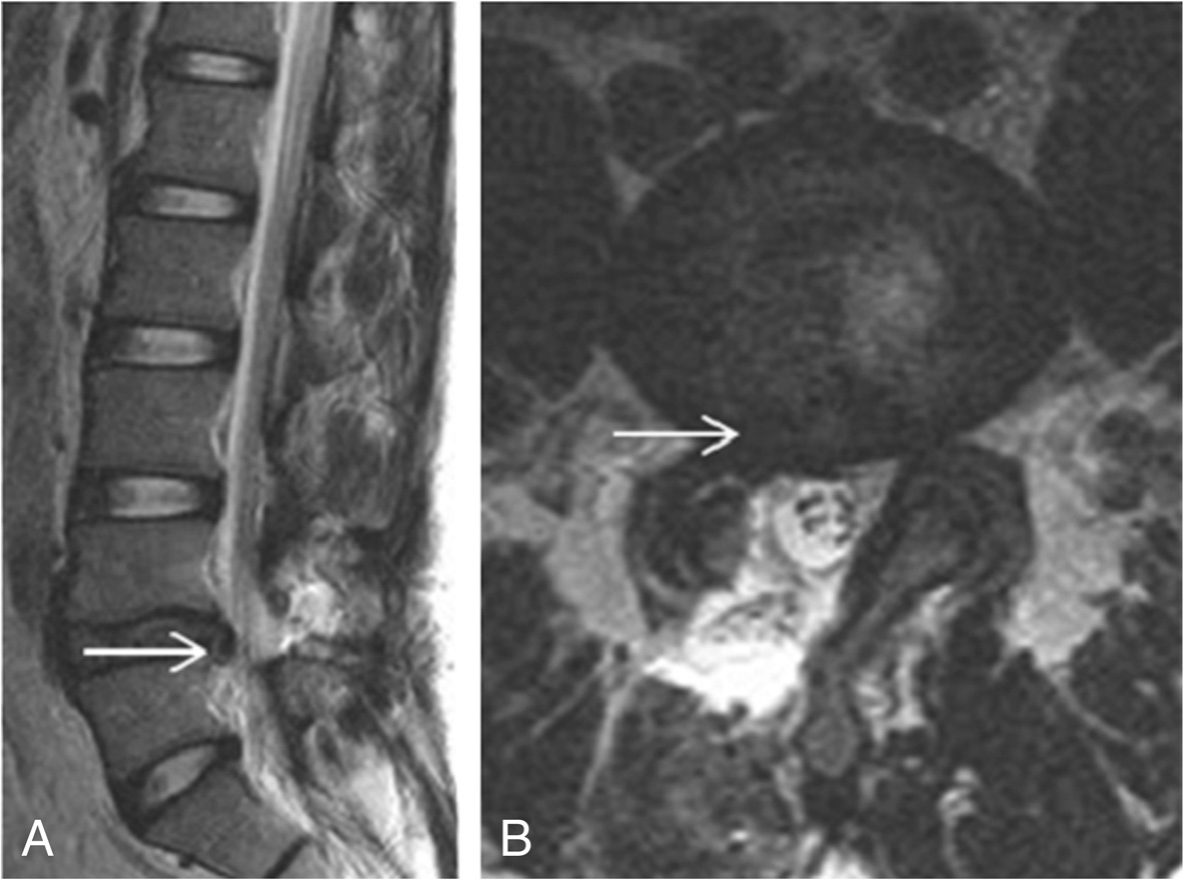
Magnetic resonance images of persistent herniated disc with dural sac compression. This patient had right buttock and leg pain for 3 months, and the symptoms persisted after right L4–5 discectomy. Postoperative a sagittal and b cross-sectional magnetic resonance imaging revealed persistent herniated disc with dural sac compression (*arrows*)
